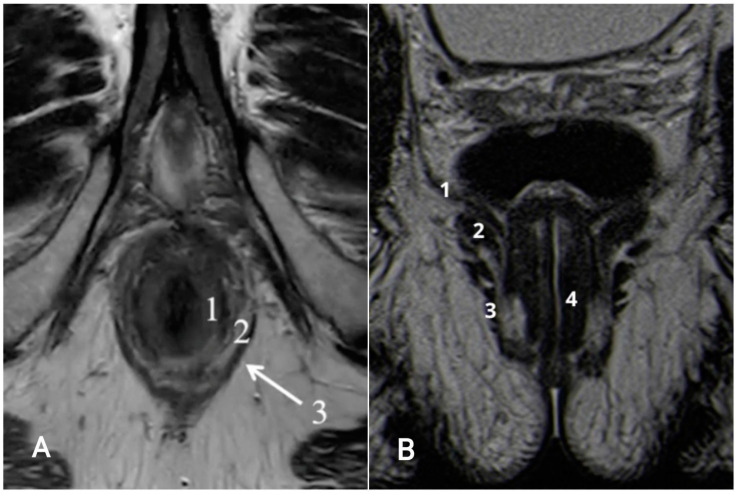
(A) Anatomy of the anal canal. T2-weighted axial MR image: 1 = internal sphincter (low–intermediate signal), 2 = fatty intersphincteric space (high signal), 3 = external sphincter (low signal, white arrow); (B) T2-weighted coronal MR image: 1 = levator ani muscle, 2 = puborectalis muscle, 3 = external sphincter, 4 = internal sphincter.
